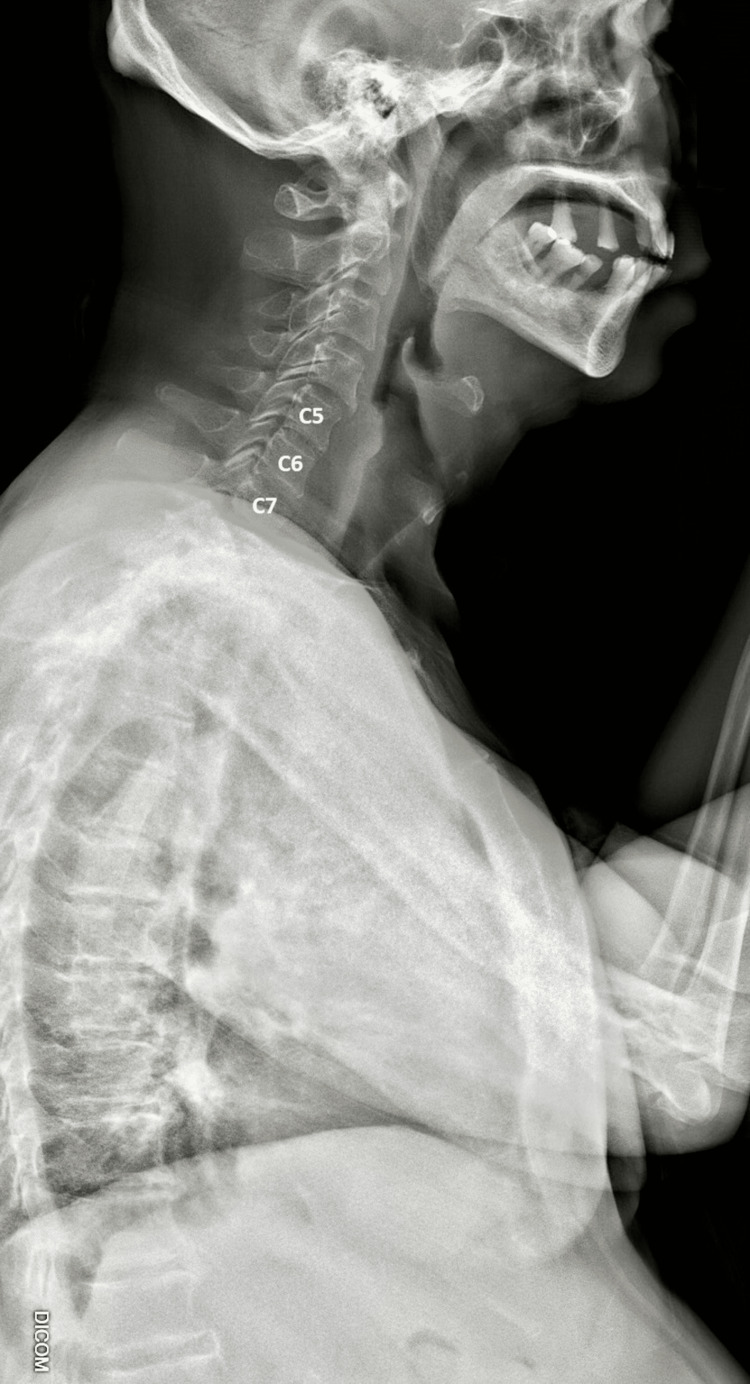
Lateral spinal radiograph Spondylosis is evident from the marginal anterior osteophytes seen in the cervical, thoracic, and lumbar spine. The disc height is mildly reduced at the C5/6 and C6/7 levels.
